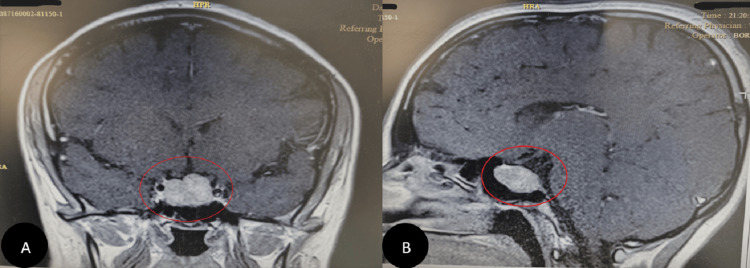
Brain MRI Finally, a pituitary adenoma was diagnosed using a Brain MRI as the cause of Cushing’s disease symptoms (A and B).
